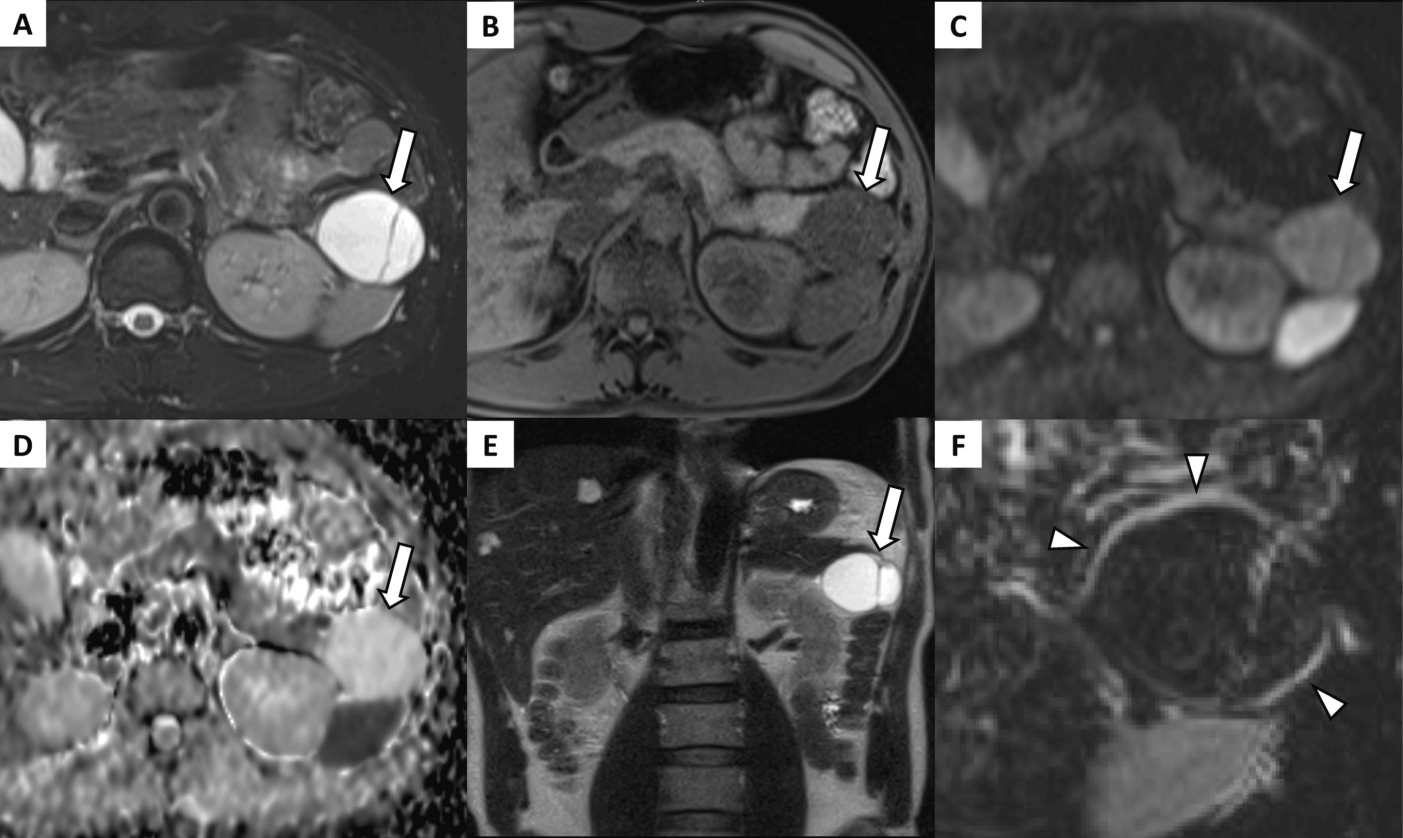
Magnetic resonance imaging findings (A) The cystic components show high intensity on fat-suppressed T2-weighted imaging with fluid-fluid levels and (B) low intensity on fat-suppressed T1-weighted imaging (arrows). (C,D) There is no diffusion restriction within the cyst (C shows diffusion-weighted imaging, and D shows the apparent diffusion coefficient map) (arrows). (E) A coronal image using half-Fourier acquisition single-shot turbo spin echo sequence (arrow). (F) A subtraction image made by subtracting superparamagnetic iron oxide (SPIO)-enhanced fat-suppressed T2-weighted imaging from pre-enhanced fat-suppressed T2-weighted imaging. The solid components show high intensity, which means a signal reduction on SPIO-enhanced fat-suppressed T2-weighted imaging, indicating SPIO uptake (arrowheads).
